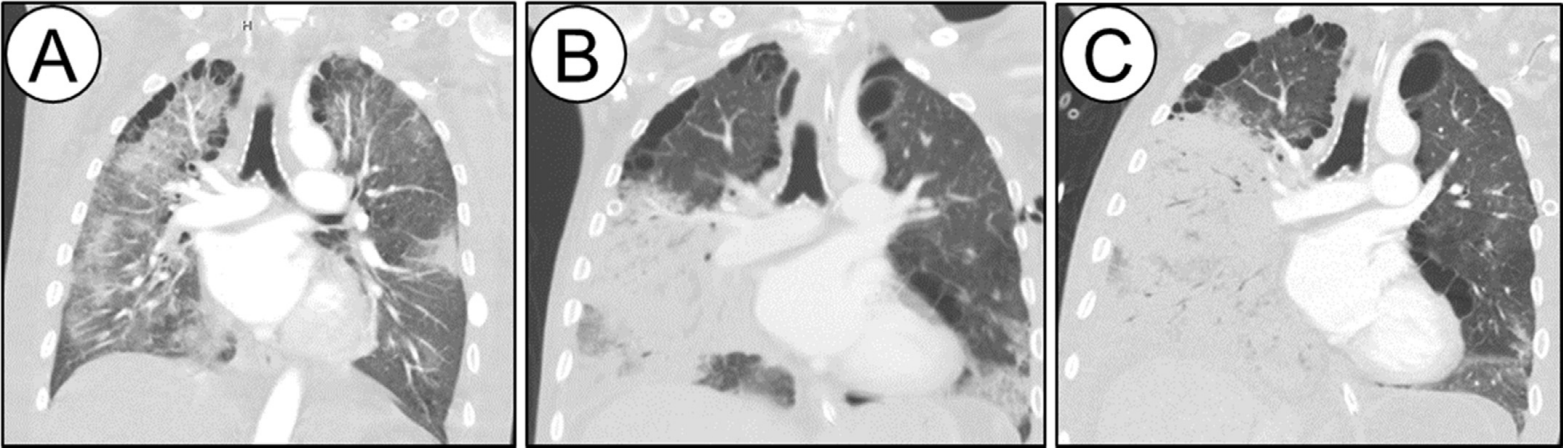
Coronal images (slices) from CT of chest scans on days 1 (A), 16 (B), and 23 (C) of hospitalization demonstrating the progression of the infiltrates in the right lung.
